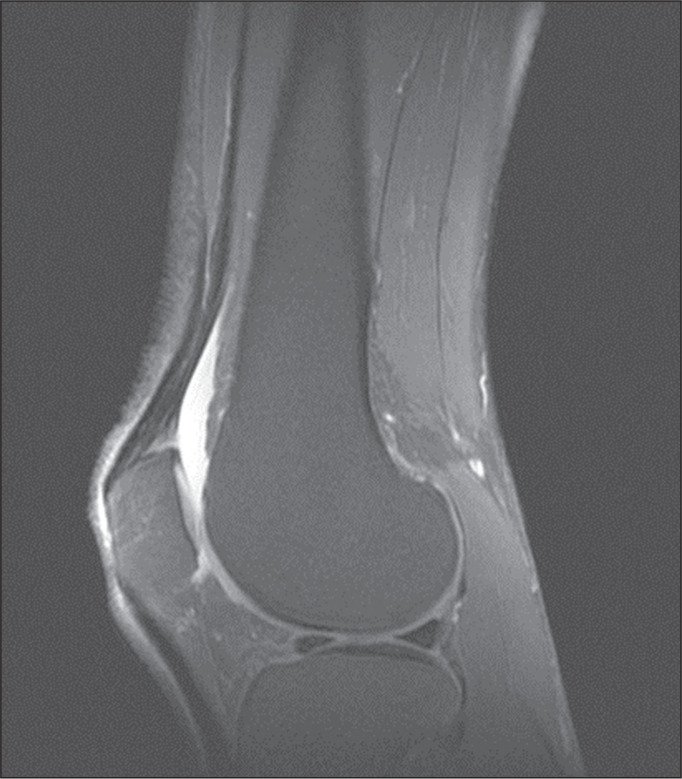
MRI of the knee, showing joint effusion.
